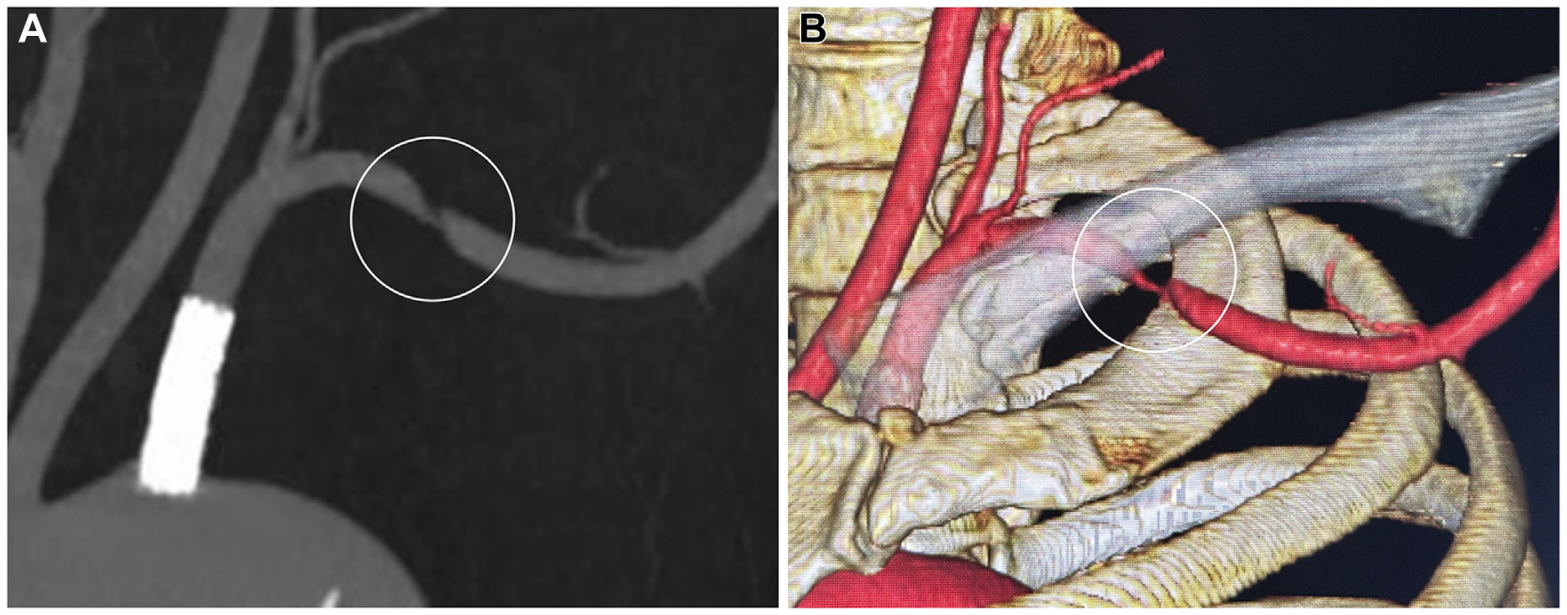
Computed tomography (CT) angiography with the arm elevated showing stenosis of the subclavian artery at the costoclavicular space.
